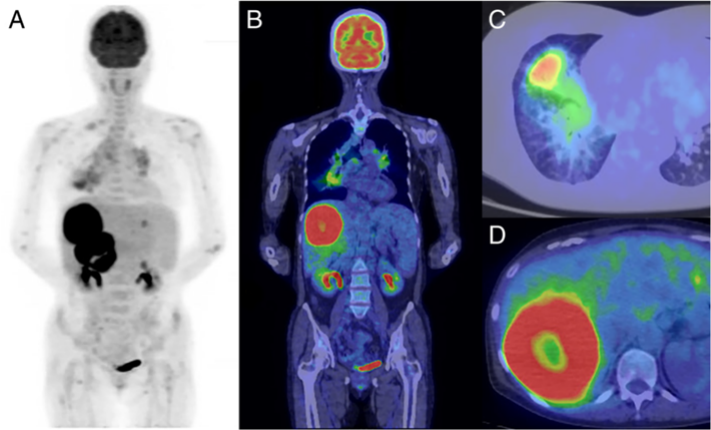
Pre-therapy FDG PET/CT images in a 54-year-old female with sarcoidosis–lymphoma syndrome. Whole-body scan of FDG PET/CT was performed at 60 min after 290.7 MBq of FDG injection. (a MIP; b. coronal fusion; c-d. axial fusion) In the liver, there were intensive ring-form uptakes in the tumors, with maximum standard uptake values (SUVmax) of 18.3 in S5 and 19.5 in S7. The faint right middle and lower lobe consolidations had moderate FDG uptake (SUVmax, 4.6), whereas the multiple bilateral hilar and mediastinal lymphadenopathies showed mild FDG uptake (SUVmax, 2.7). *FDG* 18-fluorodeoxyglucose, *PET* positive emission tomography, *CT* computed tomography, *MIP* maximum intensity projection
